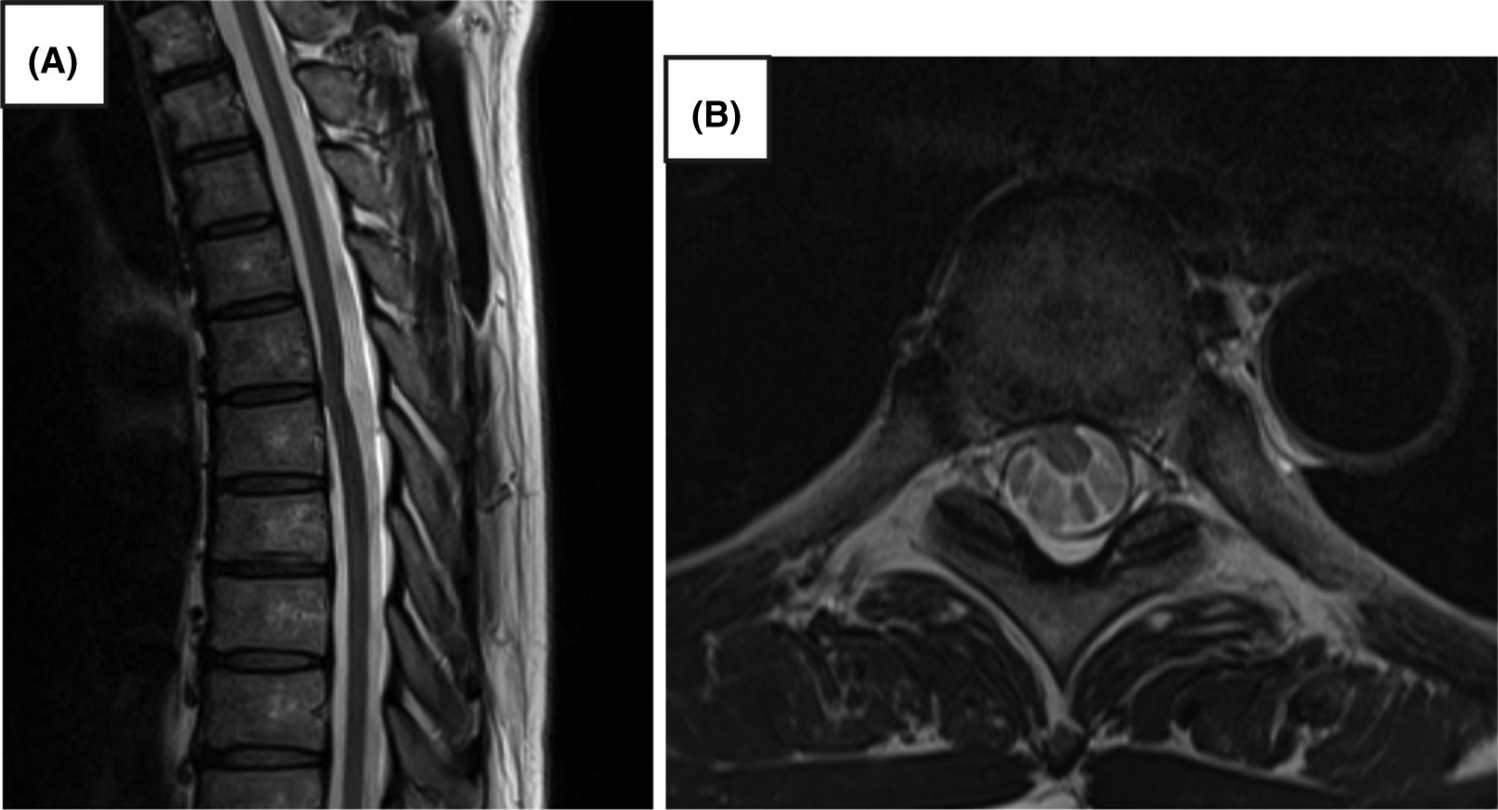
Preoperative magnetic resonance imaging (MRI). (A) Sagittal T2‐weighted MRI showing anterior deviation and flexion of the spinal cord at the Th6/7 level and loss of the spinal fluid space anterior to the spinal cord. (B) Axial T2‐weighted MRI showing ventral deviation of the spinal cord at the Th6/7 level.
